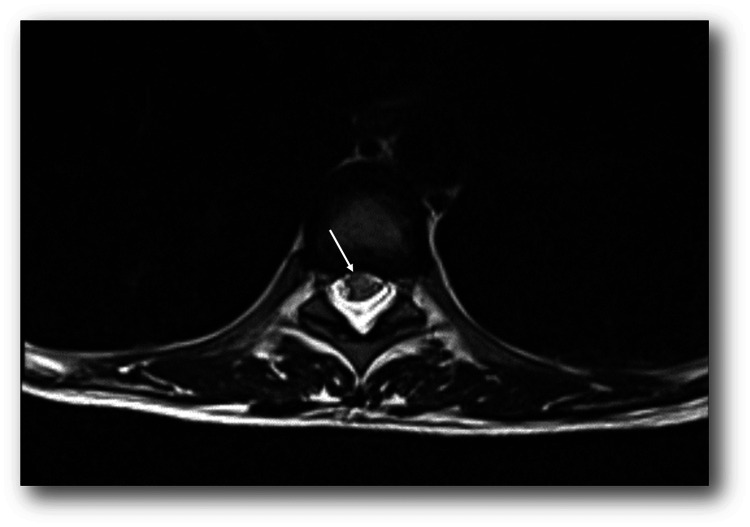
Cross-sectional T2-weighted image of the spinal cord at the T6 level showing high signal intensity (white arrow).
